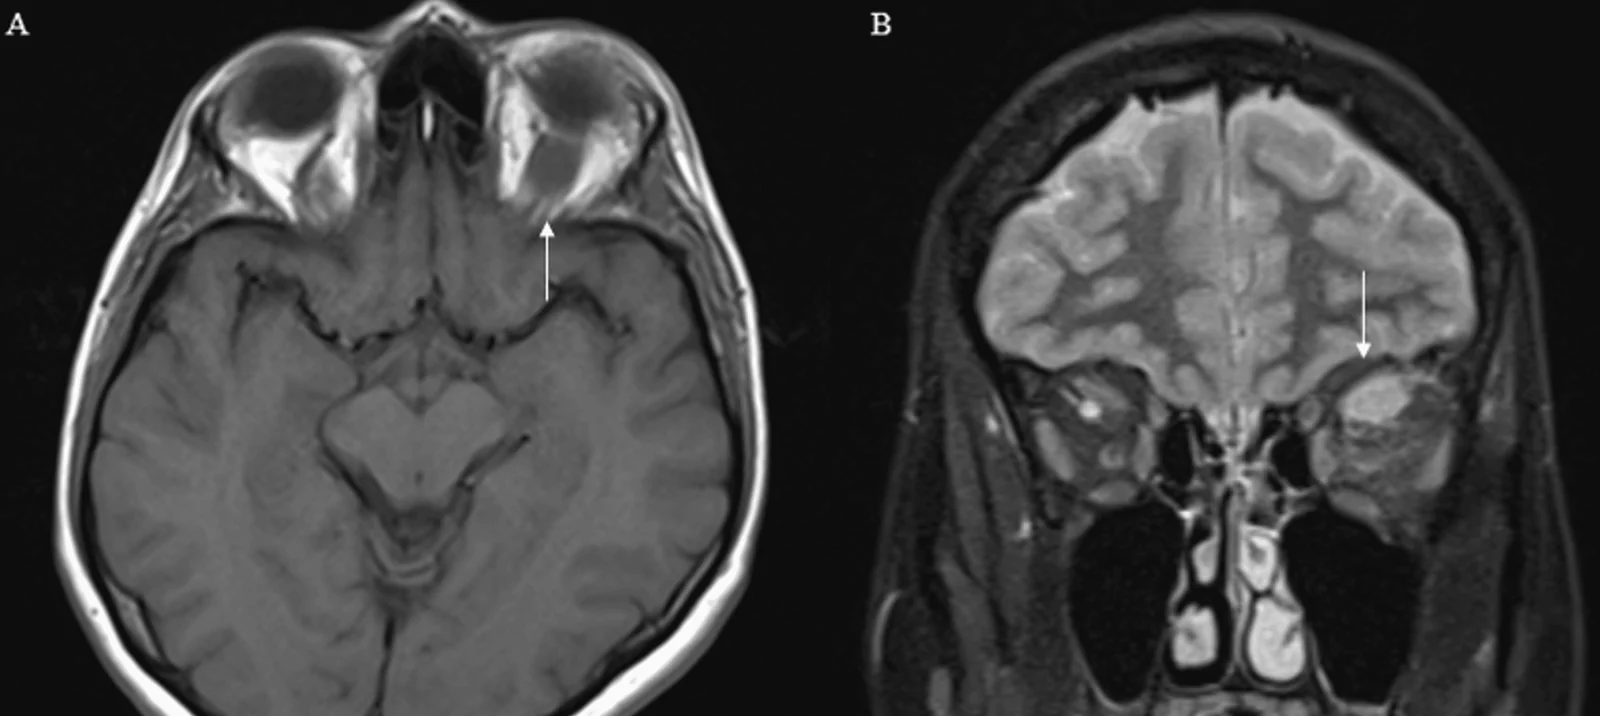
Orbital MRI (A) axial T1-weighted image and (B) coronal T2-weighted fat-suppressed image, showing fusiform enlargement of the left superior rectus muscle (arrows) consistent with active inflammation. The thickness of the superior rectus muscle was 7.8 mm, while the thickness of the contralateral muscle was 3.2 mm. No orbital abscess, optic nerve involvement, intracranial extension, or associated paranasal sinus disease was identified.
